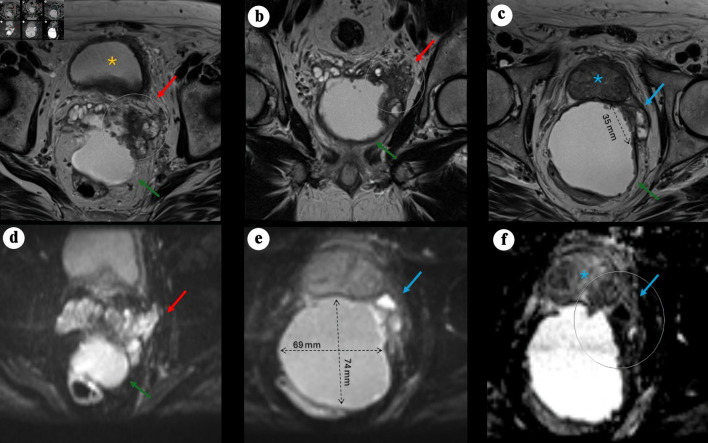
Pelvic MRI demonstrating a large cystic pelvic mass with features suggestive of malignancy. (a) Axial T2-weighted image showing a large, predominantly cystic mass (green arrow) situated between the rectum and the posterior left peripheral zone of the bladder (yellow asterisk), associated with a severely distorted left SV (red arrow) demonstrating hypoattenuation, suspected to originate from a left SV mass, with rim enhancement and peripheral nodularity (arrow). (b) Coronal view showing the mass located posteriorly and extending caudally. (c) Axial view demonstrating an additional smaller cystic focus (3.6 × 1.4 cm) between the left posterolateral mid-gland and the main lesion. Irregular T2-hypointense tissue extends between the left inferior SV, the cystic mass, and the left posterolateral base of the prostate, suspicious for malignancy (PI-RADS 5) with extraprostatic extension. (d) Axial DWI view of the mass. (e) Axial DWI showing marked diffusion restriction within the prostatic lesion and along the cyst lining (69 × 74 mm process). (f) Axial apparent diffusion coefficient (ADC) map demonstrating corresponding low signal. MRI: magnetic resonance imaging; PI-RADS: Prostate Imaging–Reporting and Data System; SV: seminal vesicle; DWI: diffusion-weighted imaging.
